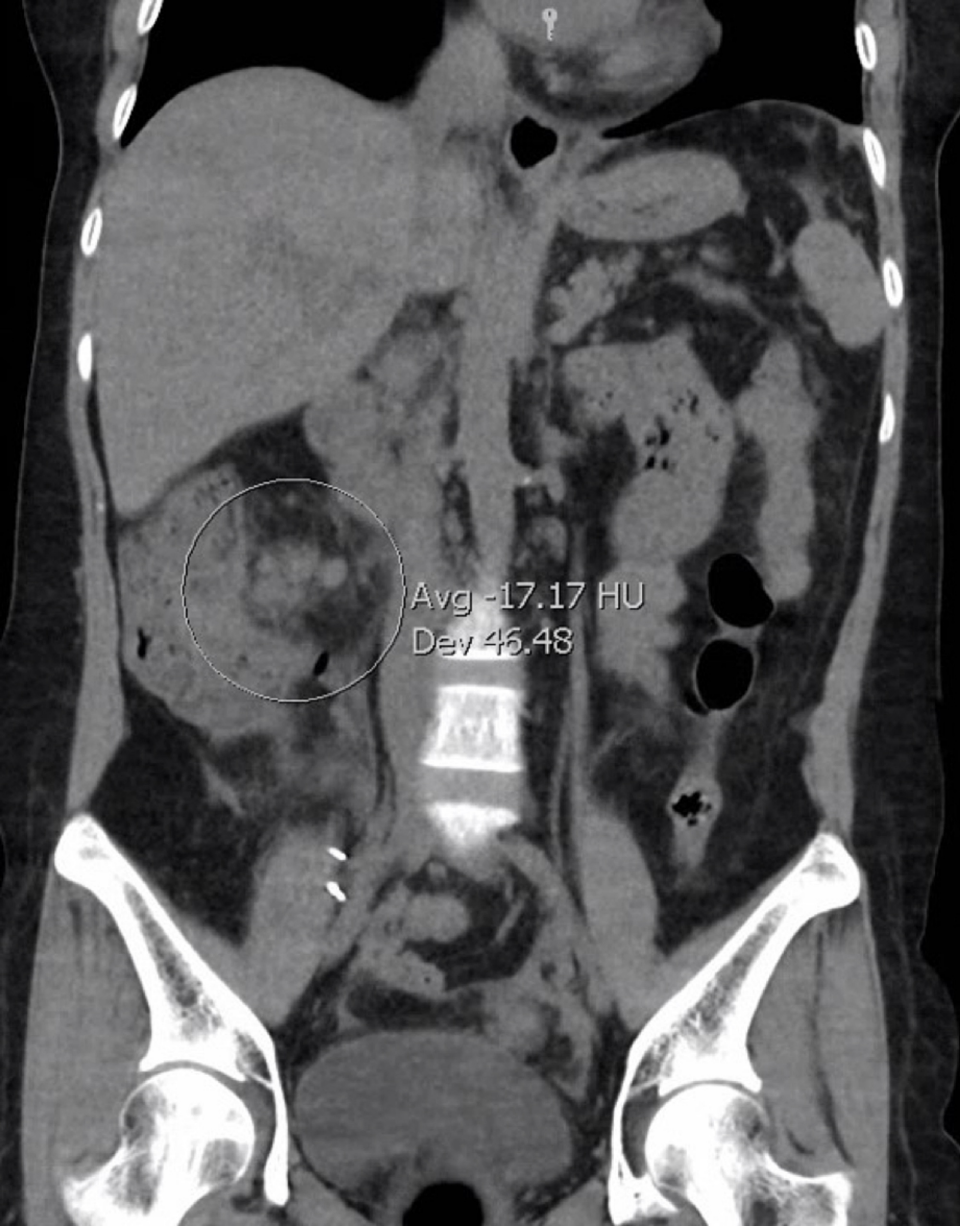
CT abdomen/pelvis showed marked thickening of the terminal ileum and prominent ileocolic lymphadenopathy.
